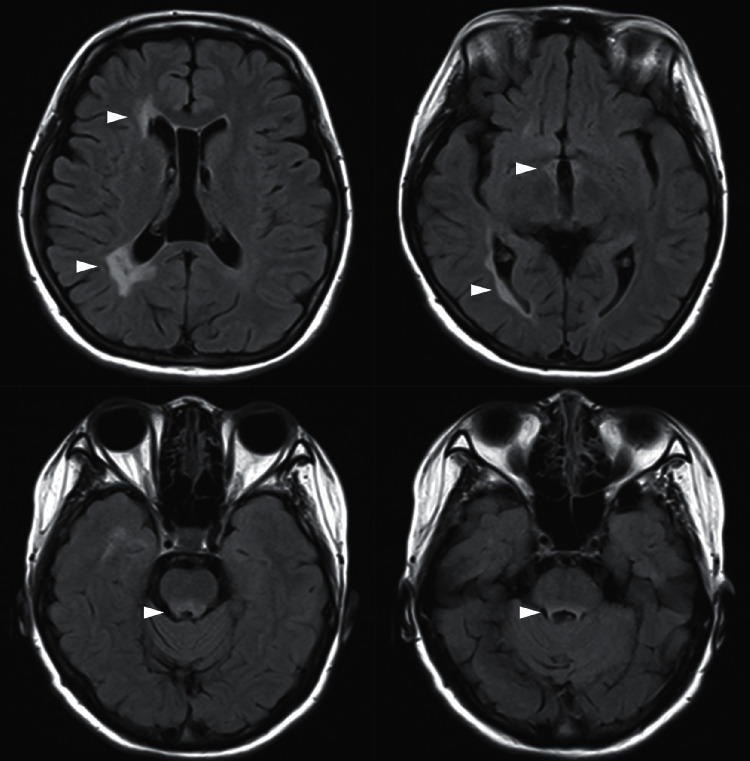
Axial FLAIR images from a 28-year-old female with NMO, disease duration of 2 years, with bilateral visual impairment and general weakness, showing high signal white matter lesions in typical periependymal regions (arrowhead).
